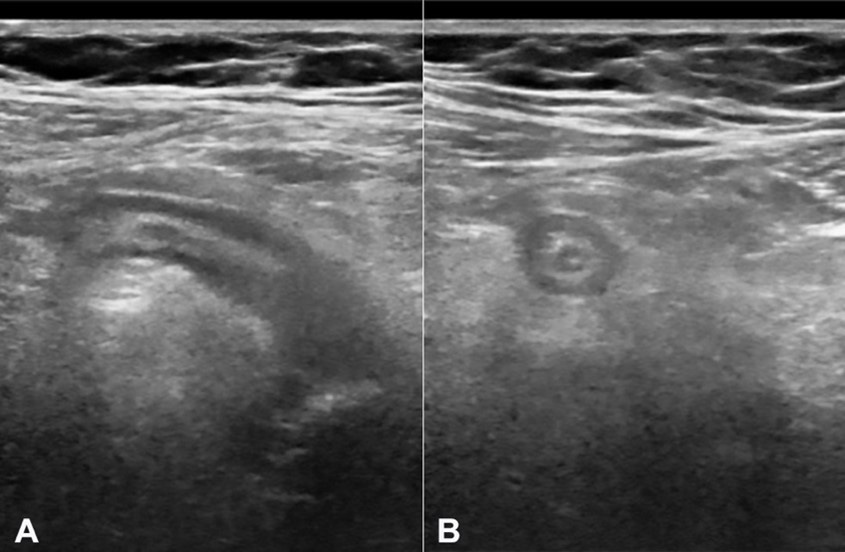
Abdominal ultrasound. Distended ileocecal appendix (13mm in diameter), with hypoechoic lumen, parietal thickening, and diffuse hyperreflectivity of the surrounding fat (A and B).
